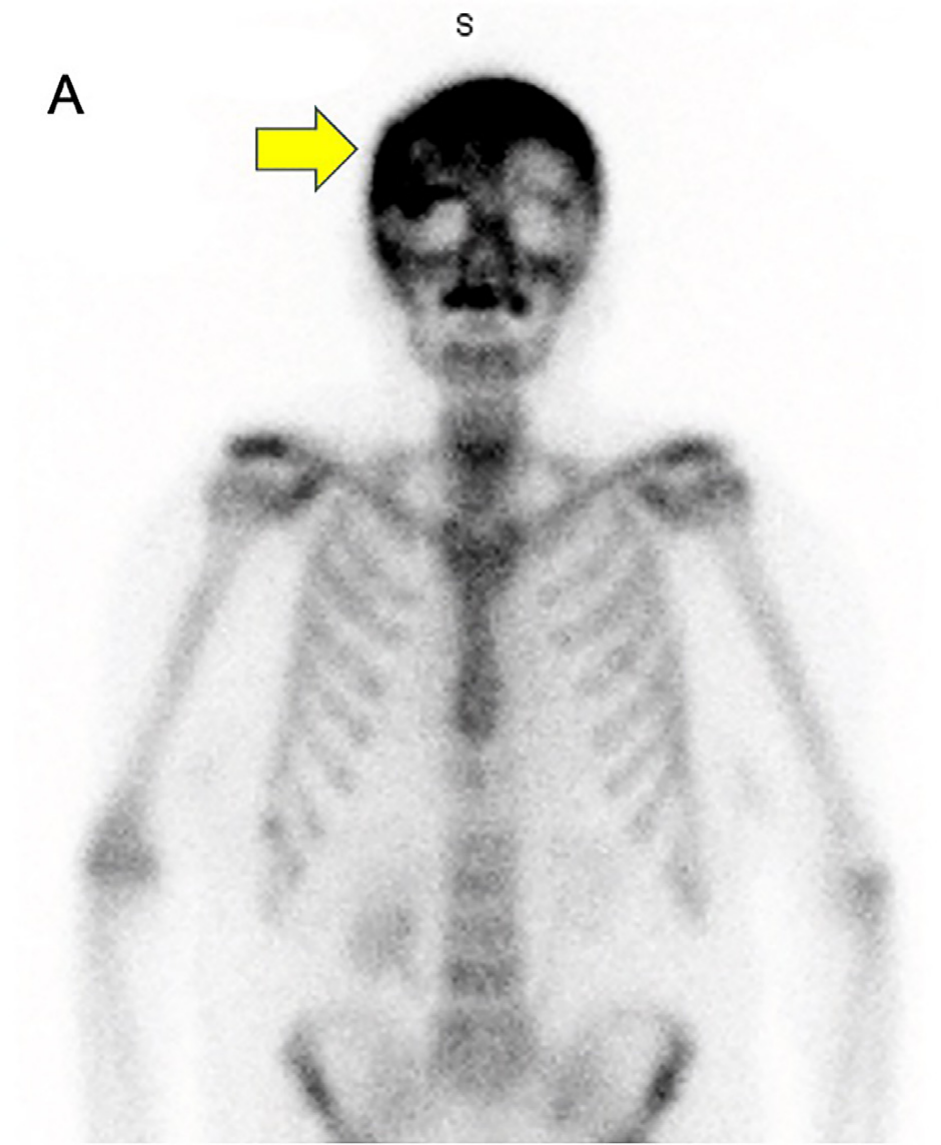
Whole body bone scintigraphy, anterior view. (A) Increased radiotracer uptake in the cranium, especially on the right frontal prominence that coincides with the lucent area found on CT (yellow arrow).
